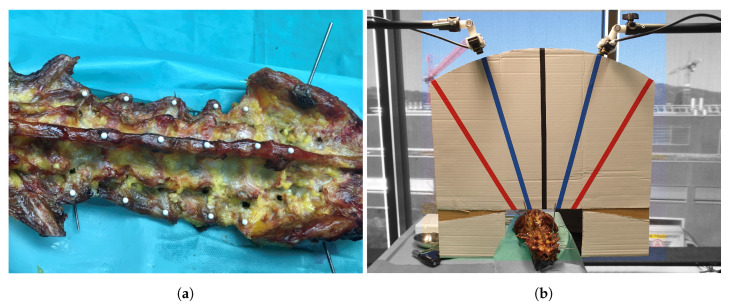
Setup for target registration error (TRE). (a) TRE were determined by push-pins, detectable in RGB-D frames and postoperative CT, inserted into both transverse processes and the spinous process of levels L1–L5. (b) Custom-made cardboard rule to set up standardized TRE viewpoints for RGB-D sensors at 0° (black), ±15° (blue) and ±30° (red).
